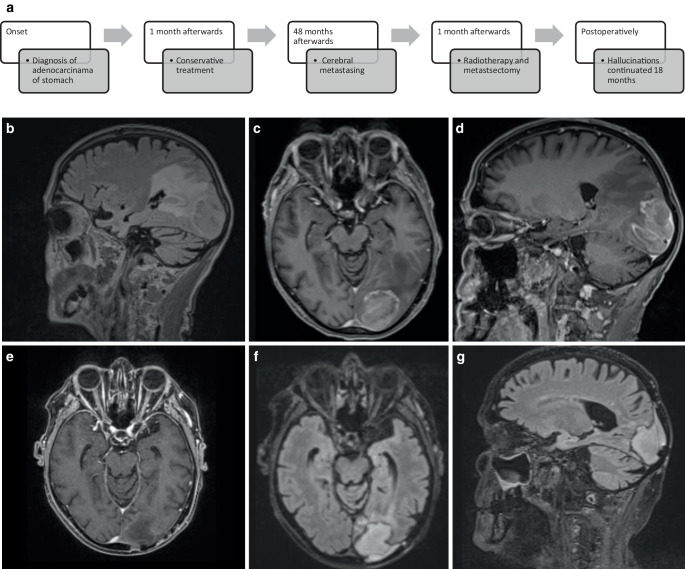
a Timeline case 1. b, c, d MRI images at time of diagnosis. b Sagittal T2 fluid-attenuated inversion recovery (FLAIR)-weighted sequence depicts a left occipital paramedian dural iso- to hypointense lesion. Axial (c) and sagittal (d) T1-weighted contrast-enhanced sequences show a slightly hypointense, ring-enhancing, and partially cystic mass with marked perilesional edema. There was a minor midline shift. e, f, g MRI images at 7 months after metastasis resection with subsequent postoperative changes. T1-weighted contrast-enhanced axial image (e), T2 FLAIR-weighted axial image (f), and sagittal image (g) show the resection cavity, with slight enhancement at the margins, and the adjacent sub-/epidural collection in the T2-weighted sequence
